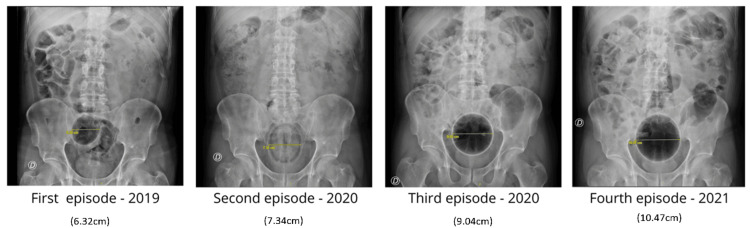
Sequential abdominal X-rays from each episode.
